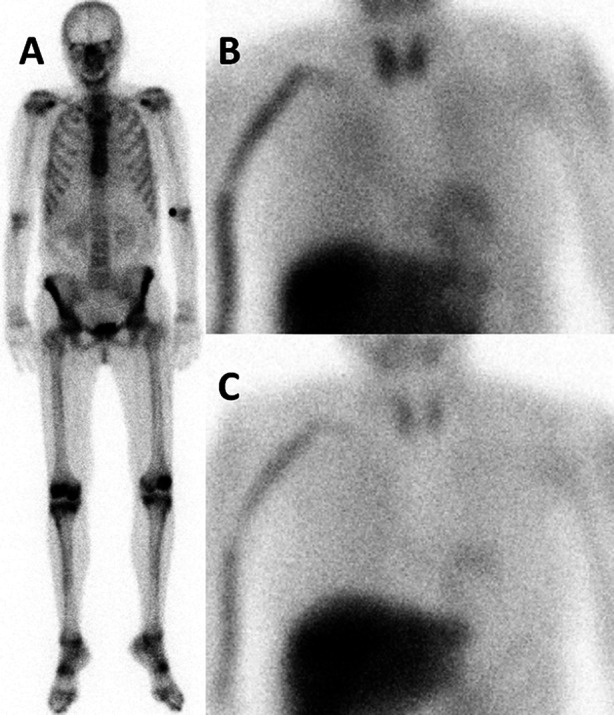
(A) Planar anterior view of ^99m^Tc-HDP (^99m^Technetium-hydroxymethylene diphosphonate) scintigraphy in a middle-aged female patient with ATTRm (mutant transthyretin amyloidosis), showing physiological tracer distribution in the bones and normal excretion through the urinary tract, but no cardiac tracer accumulation. Anterior views of (B) early time point and (C) late time point ^123^I-MIBG (^123^Iodine-metaiodobenzylguanide) scintigraphy, showing a decrease in cardiac ^123^I-MIBG HMR (heart-to-mediastinum ratio; decreasing from 1.70 to 1.50, respectively, normal value in our laboratory for late HMR 2.00)
